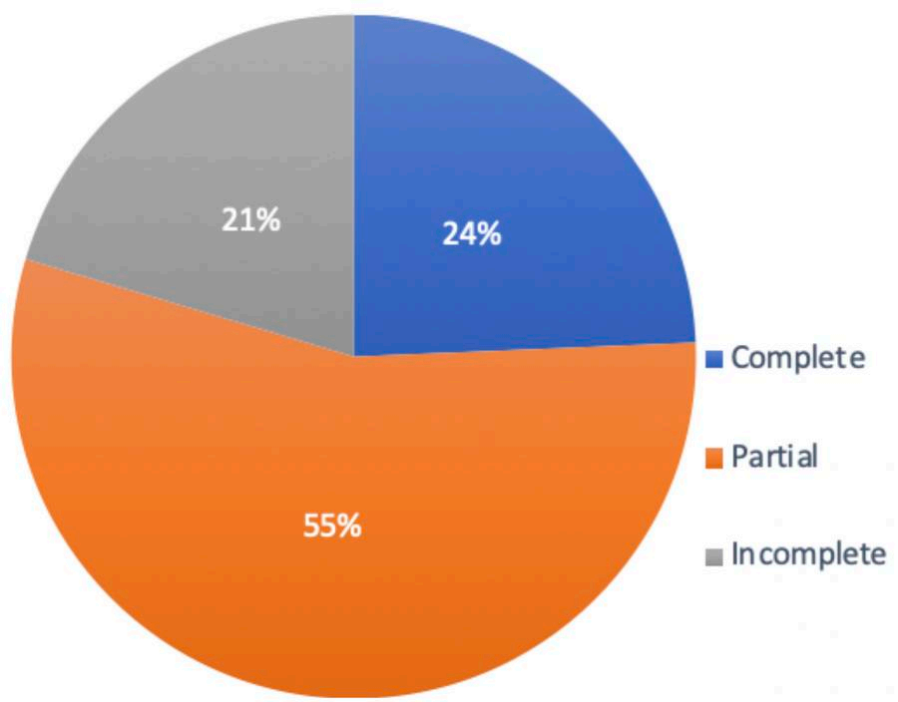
Pie chart demonstrating the incidence of a complete, partial, and incomplete circle of Willis in a south Trinidad population referred for head MRI/MRA at the San Fernando General Hospital between October 1, 2019 and September 30, 2020.
